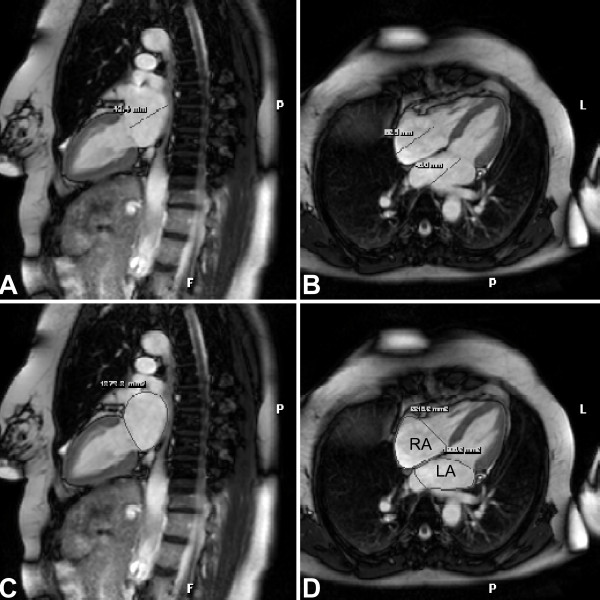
CMR images with representative measurements at atrial end-diastole. (A) Two-chamber (2 Ch) view with left atrial (LA) length measurement. (B) Apical four-chamber (4 Ch) view with LA and right atrial (RA) 4 Ch length measurement. (C) 2 Ch view with LA endocardial border tracings. (D) 4 Ch view with LA and RA endocardial border tracings. P: Posterior. L: Left.
